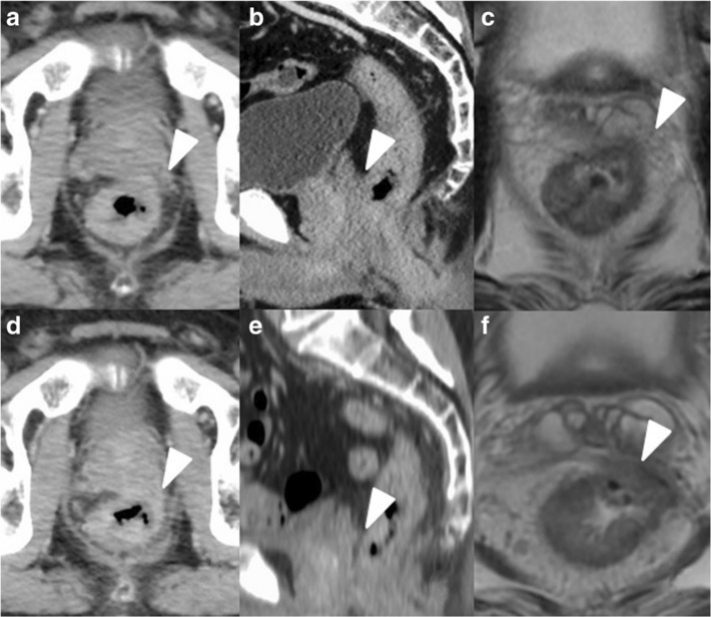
CT and MRI show the tumor invading the left seminal grand and prostate (*white arrowheads*). After CRT, the degree of invasion to adjacent organs was unchanging. a–c Pre-CRT CT, MRI image. d–f Post-CRT CT, MRI image
